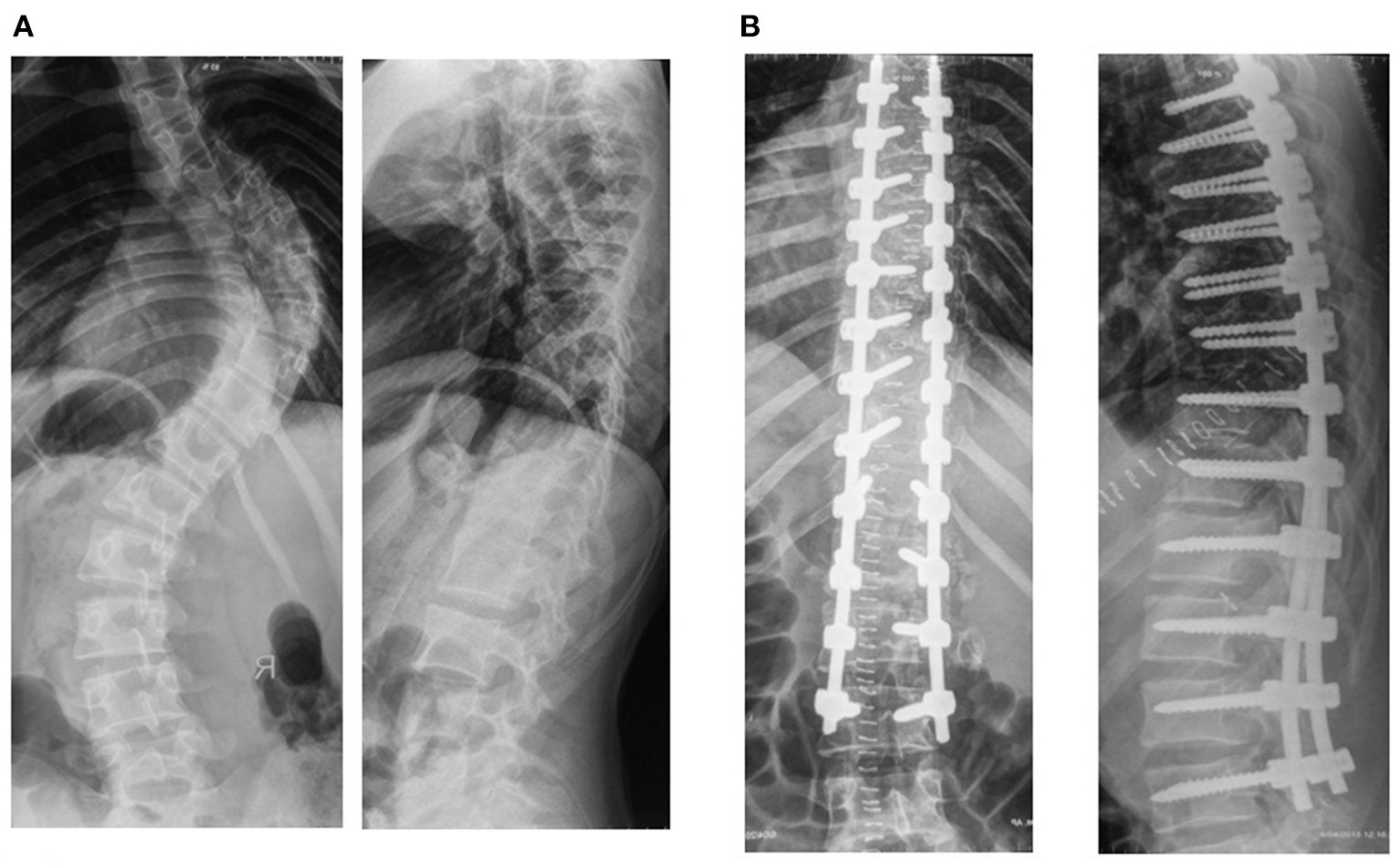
Spinal radiographs of patient T (16 years old). Idiopathic right thoracic scoliosis. (A) Preoperatively, the angle of scoliotic deformity was 86° according to Cobb, the angle of kyphosis was 13°, and the angle of lordosis was 22°. (B) Postoperatively, the angle of scoliosis was 5°, the angle of kyphosis was 22°, and the angle of lordosis was 26°.
